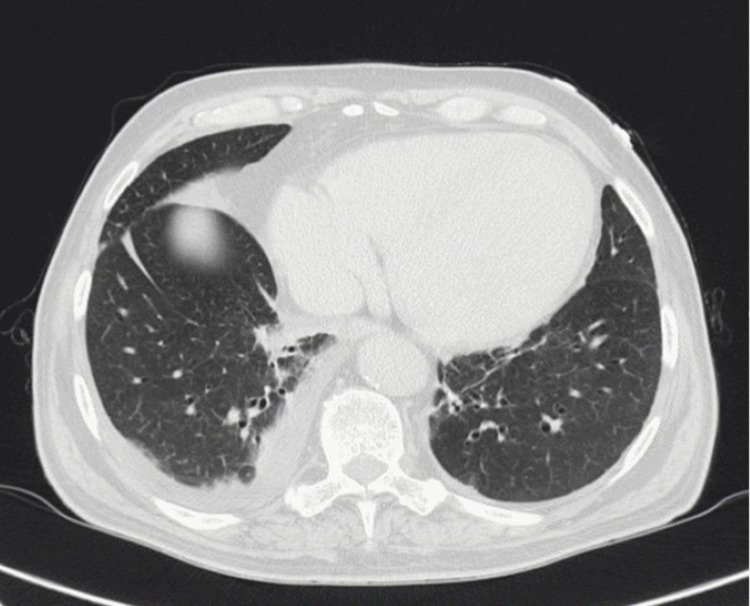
Thoracic CT scan revealing cardiomegaly and pleural effusion on the right lung
